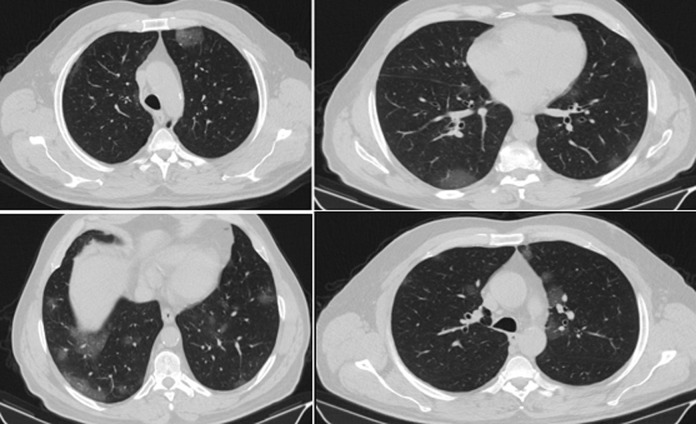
chest computed tomography; widespread, predominantly peripheral multifocal ground glass densities were observed in both lungs
